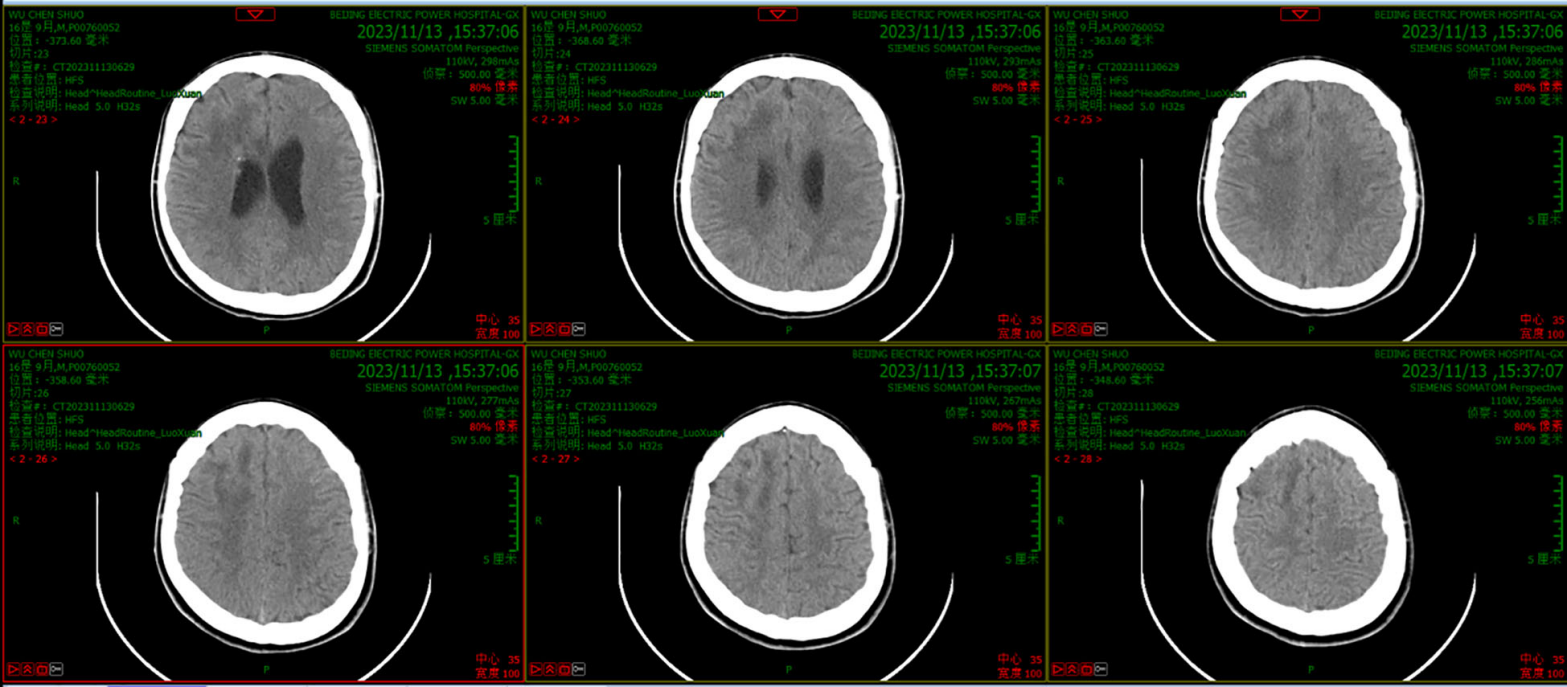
CT scanning results after treatment with the new regimen for 14 days showed that the abscess had disappeared and that there was little edema in the surrounding brain tissue.
